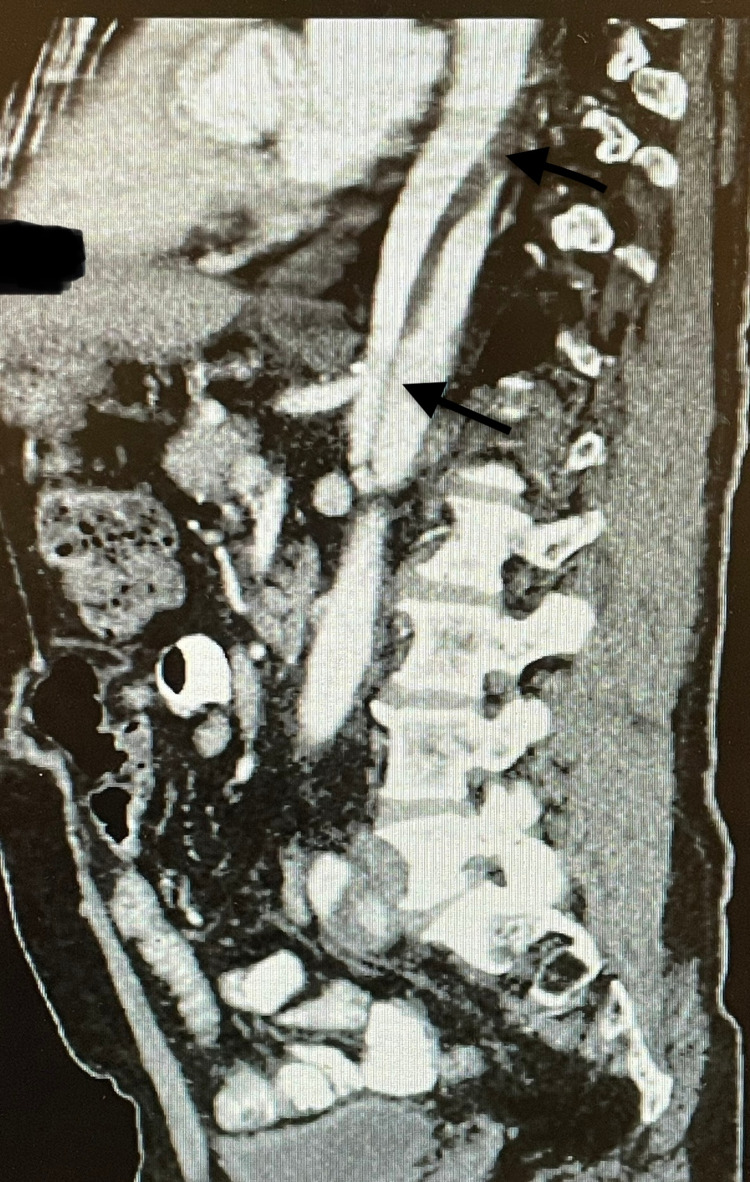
Extensive aortic dissection as seen on CT angiogram CT: computed tomography
